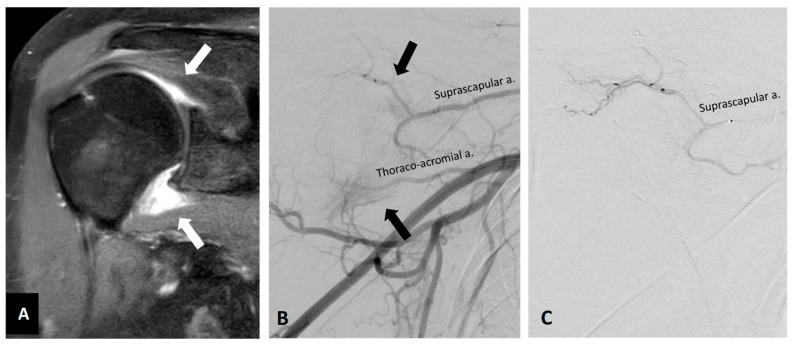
Representative pre- and postprocedural images of the right shoulder adhesive capsulitis in a 56-year-old female with chronic pain and limited range of motion. (A) Preprocedural coronal MRI (fat-saturated T1-weighted post-contrast) revealed synovium and capsule enhancement (white arrows). (B) Preprocedural angiography of the right axillary artery showed angiogenesis (black arrows) over the corresponding enhanced area of MRI. (C) Postembolization angiography via thoracoacromial artery and other arteries indicated the elimination of angiogenesis after TAE. The patient’s pain and range of motion were improved after TAE.
